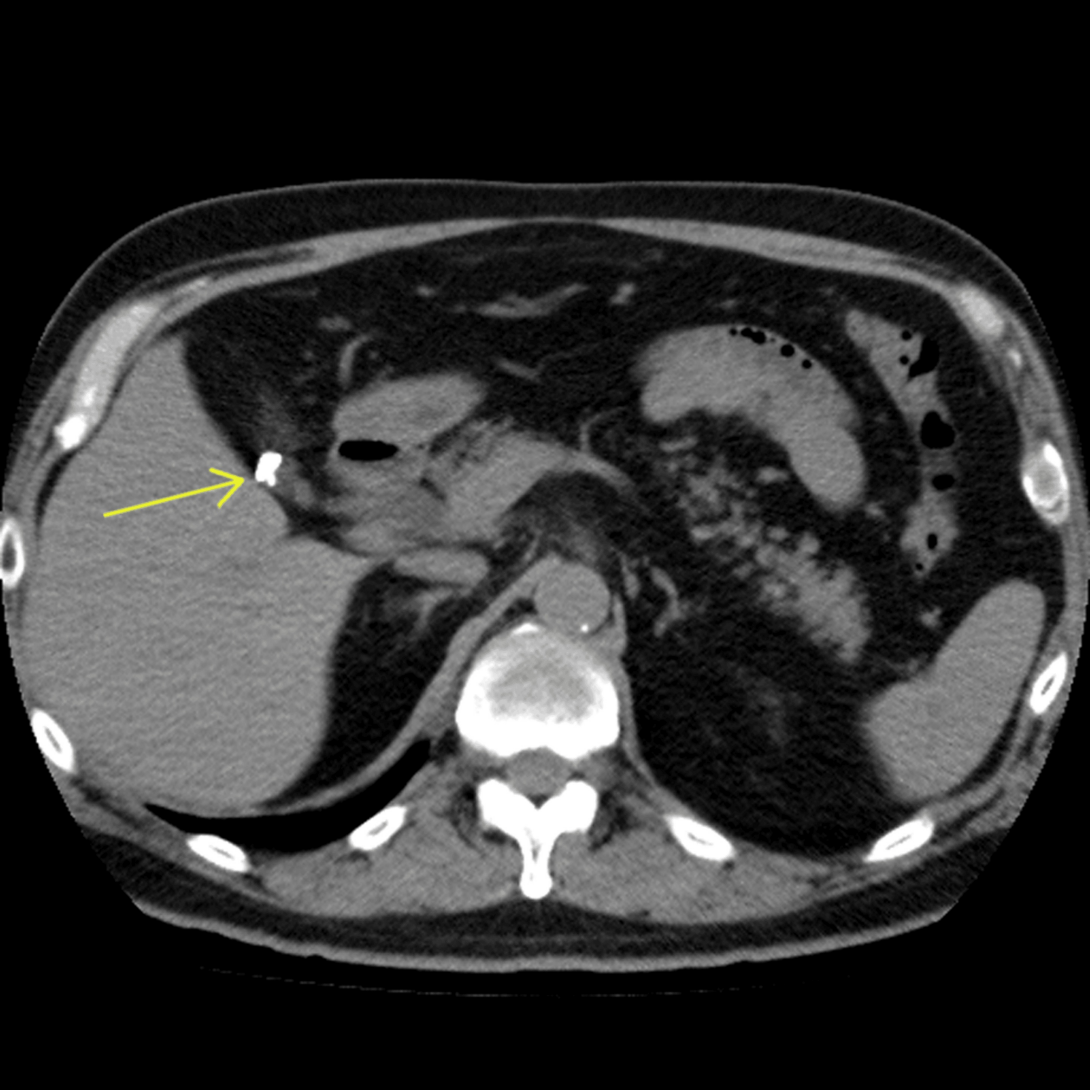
Axial CT performed 15 years prior to the patient's development of symptoms showing no evidence of clip migration and the correct placement of all surgical clips.
